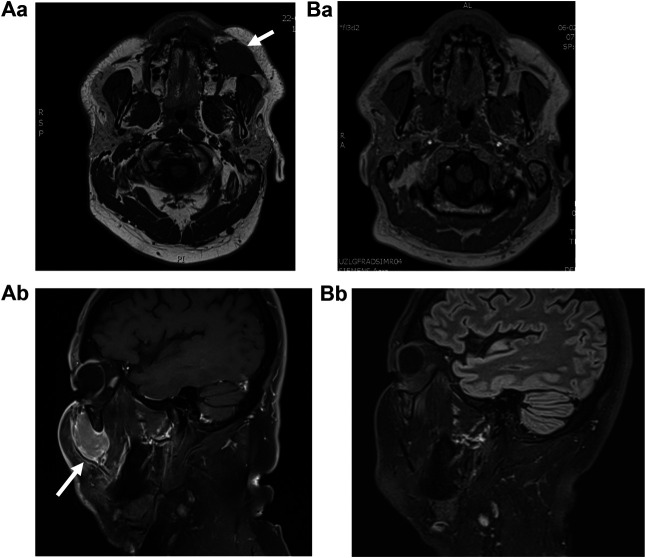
MRI (Magnetic Resonance Imaging) (A) at the moment of first presentation, depicting a volume of 28 mm × 29 mm × 42 mm (AP × ML × CC) and (B) 5.5 months later, after an incision biopsy was performed. Aa, This T2 weighted image depicts a well-described, mass of intermediate intensity at the buccinator space. Ba, T2 weighted image depicting the absence of the lesion, indicative for tumor remission. Ab, T1 weighted image with Gadolinium contrast, showing expansion to the infraorbital region. Contrastcaptation is moderate. Bb, T1 weighted image depicting the absence of the lesion.
